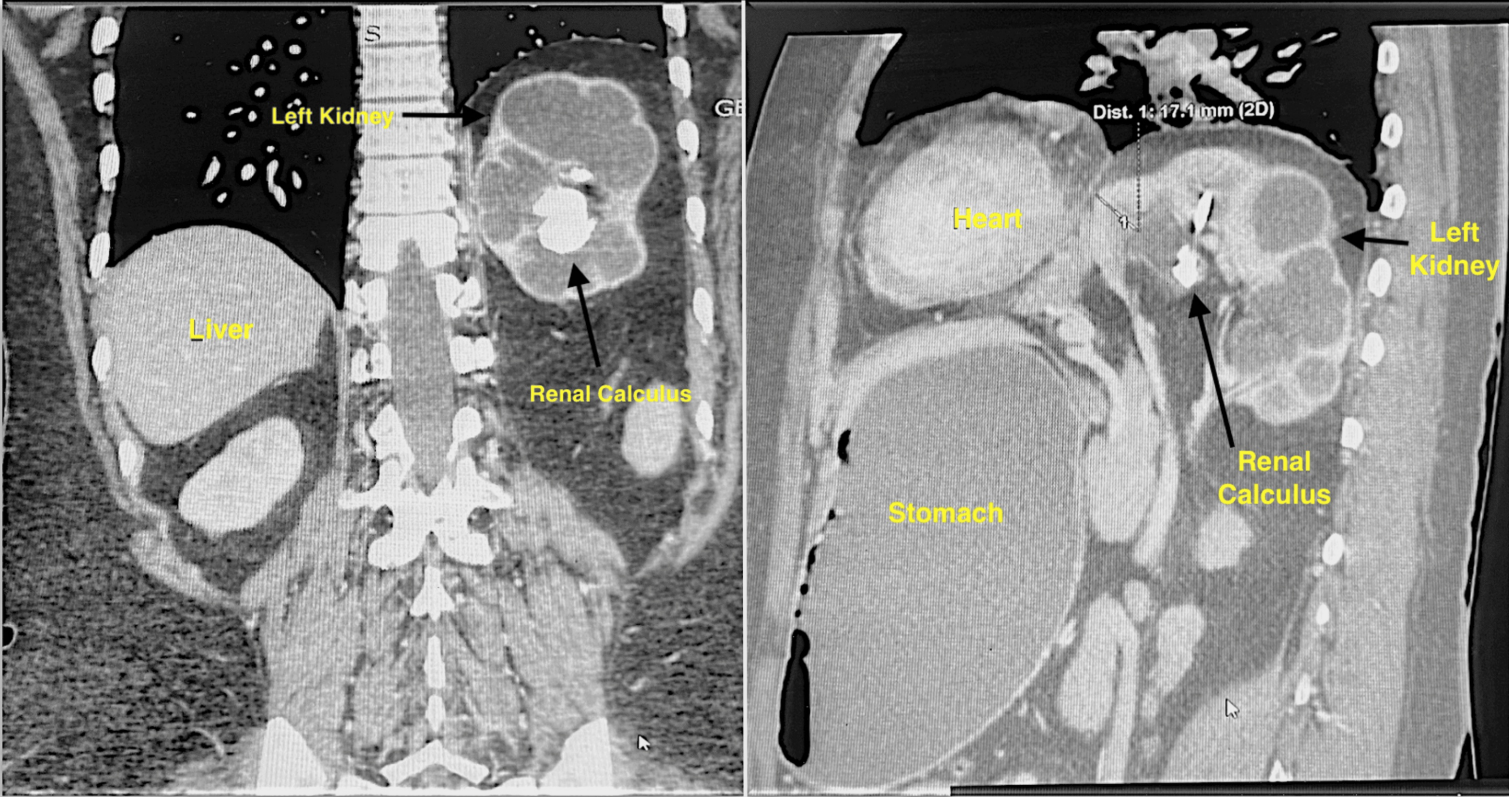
Computed tomography; coronal and sagittal sections (respectively) showing position of the left kidney relative to other organs of the body.
